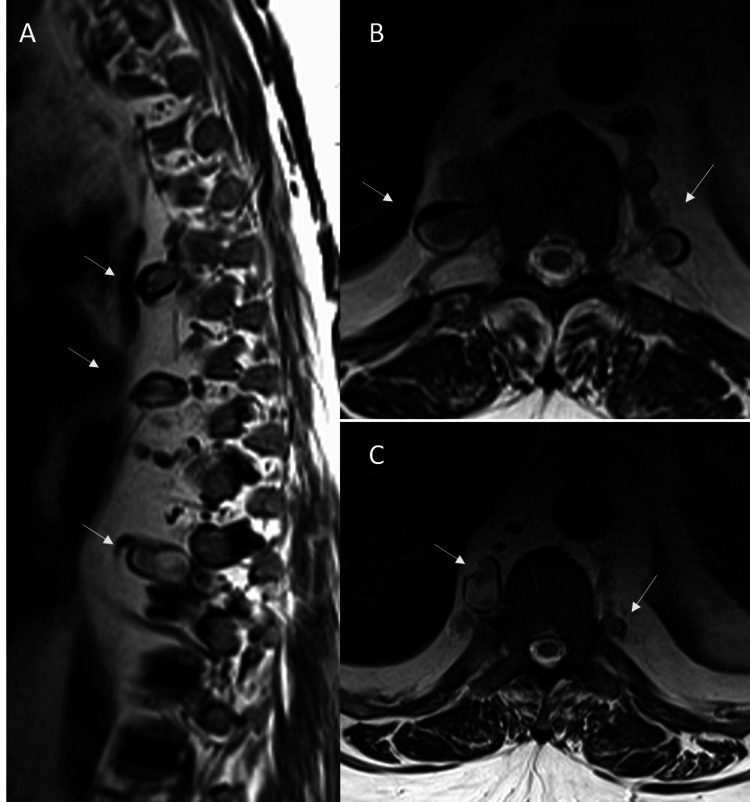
Intercostal artery aneurysmosis on chest MRI Sagittal (A) and axial (B, C) T2-weighted images reveal multiple bilateral intercostal artery aneurysms (arrows) with the standard caliber of the aorta and no evidence of any vascular malformation within the spinal canal.
